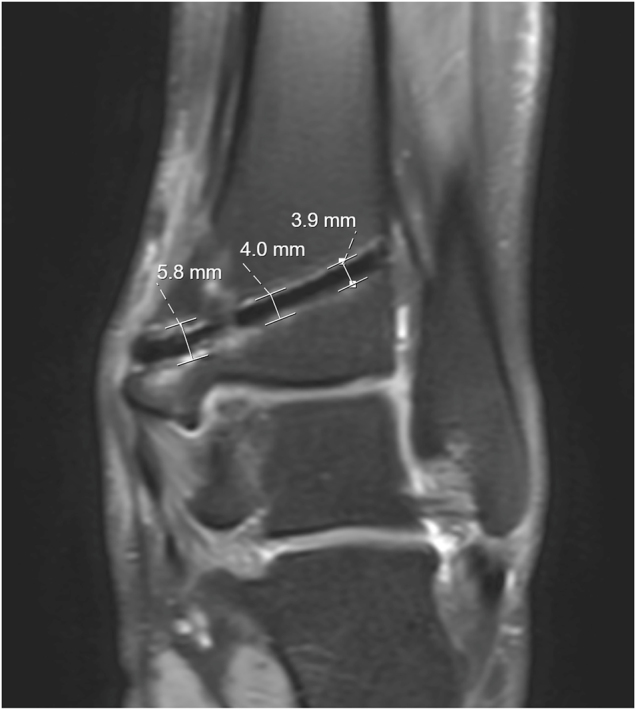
Measurement of artifact extent around the screw on a proton density weighted (PDw) coronal MR image.
